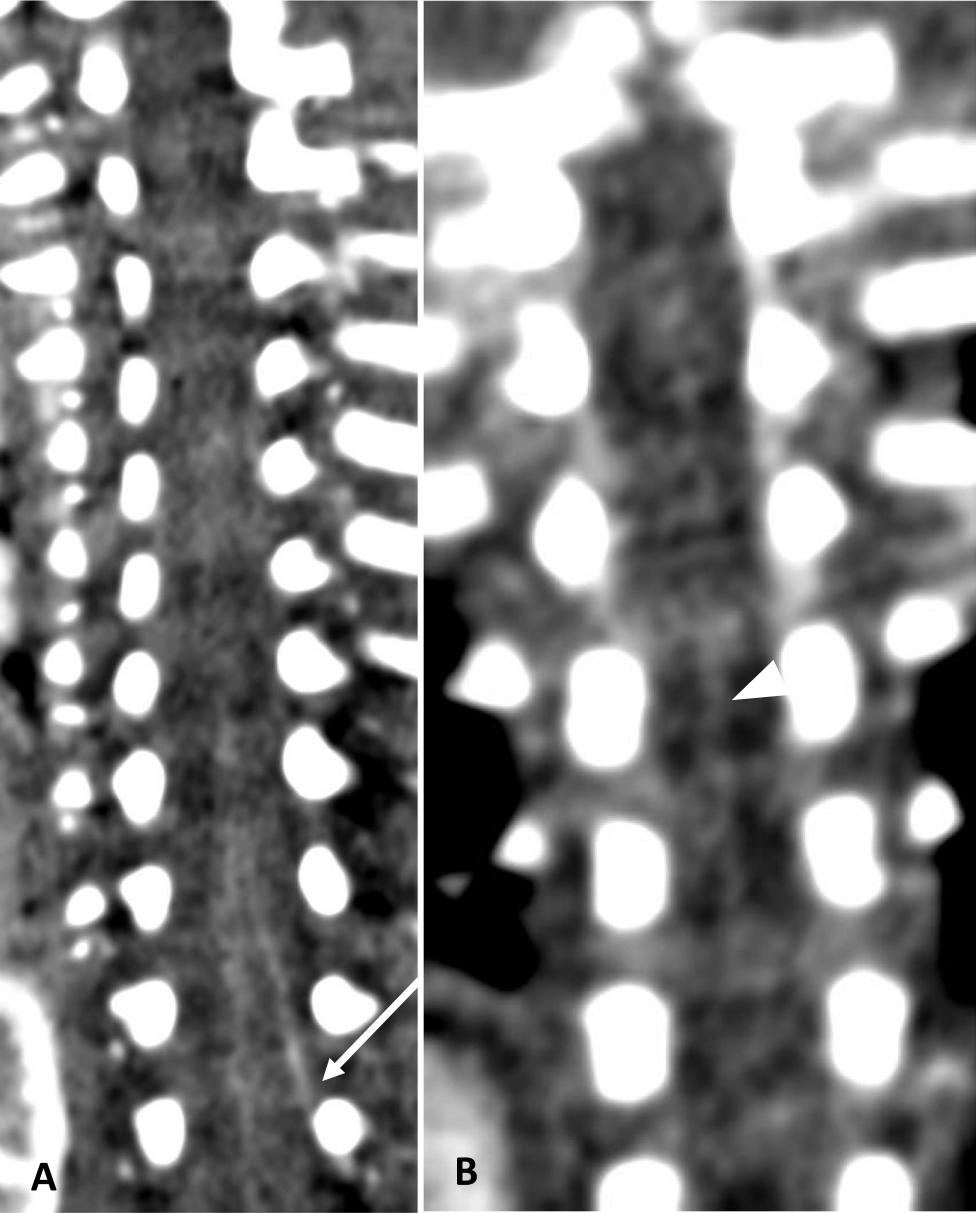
Two patients of similar age. A An 11-week-old male with a body surface area of 0.31 m^2^. Coronal CTA chest in soft tissue window. kV=70, mAs=270. The artery of Adamkiewicz can be seen arising from L2 on the left (*arrow*). B A 10-week-old female with a body surface area of 0.21 m^2^. Coronal MIP image of the CTA chest in soft tissue window. kV=70, mAs=135. The anterior spinal artery is faintly seen (*arrowhead*), but an artery of Adamkiewicz cannot be identified
